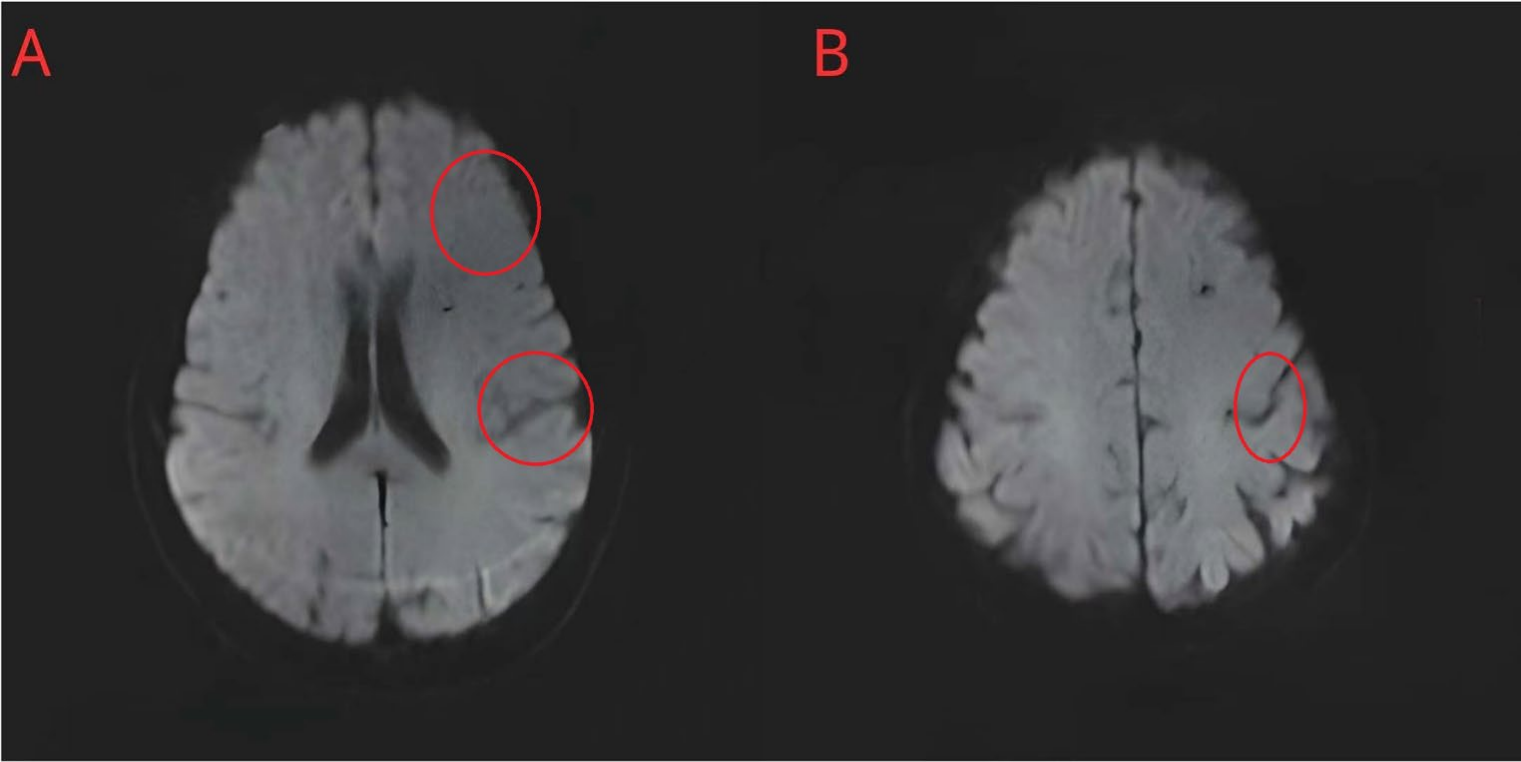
Preoperative brain MRI. The MRI shows low signal intensity in both cerebral hemispheres on DWI (red circles) without significant infarct lesions in the left frontal lobe (A) or at the junction of the left frontoparietal lobes (B). This indicates that the patient had no cerebrovascular abnormalities before thoracic surgery
